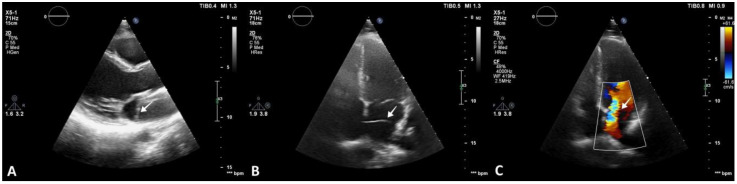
Transthoracic echocardiography revealing CTS. (A) Parasternal long-axis 2D view showing an echodense, membranous structure within the LA (arrow). (B) Apical four-chamber 2D view showing the membrane bisecting the LA (arrow). (C) Apical four-chamber 2D view and color Doppler window placed over the LA, highlighting turbulent flow across the membrane (arrow).
